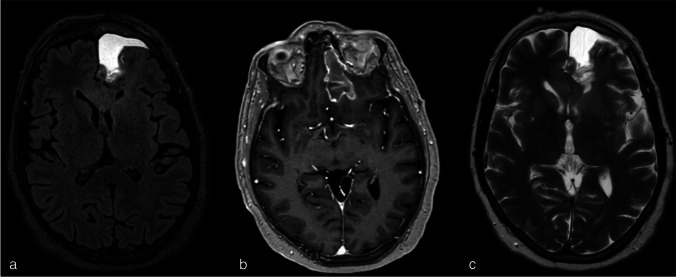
Postoperative MRI cerebrum: a T2-weighted imaging, b contrast-enhanced T1-weighted imaging, c FLAIR
